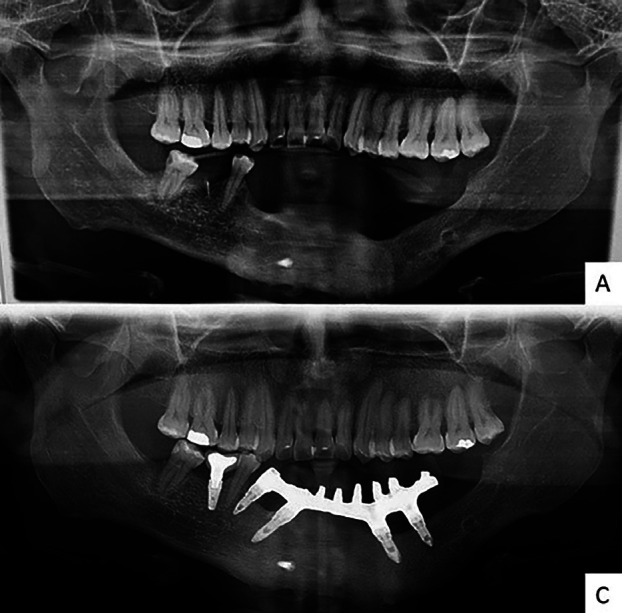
Panoramic radiographs showing A) follow-up of 120 months with bone remodeling without residual lesion. B) Five osseointegrated dental implants in the mandibular region and radiopaque material compatible with metallic bar of protocol prosthesis.
